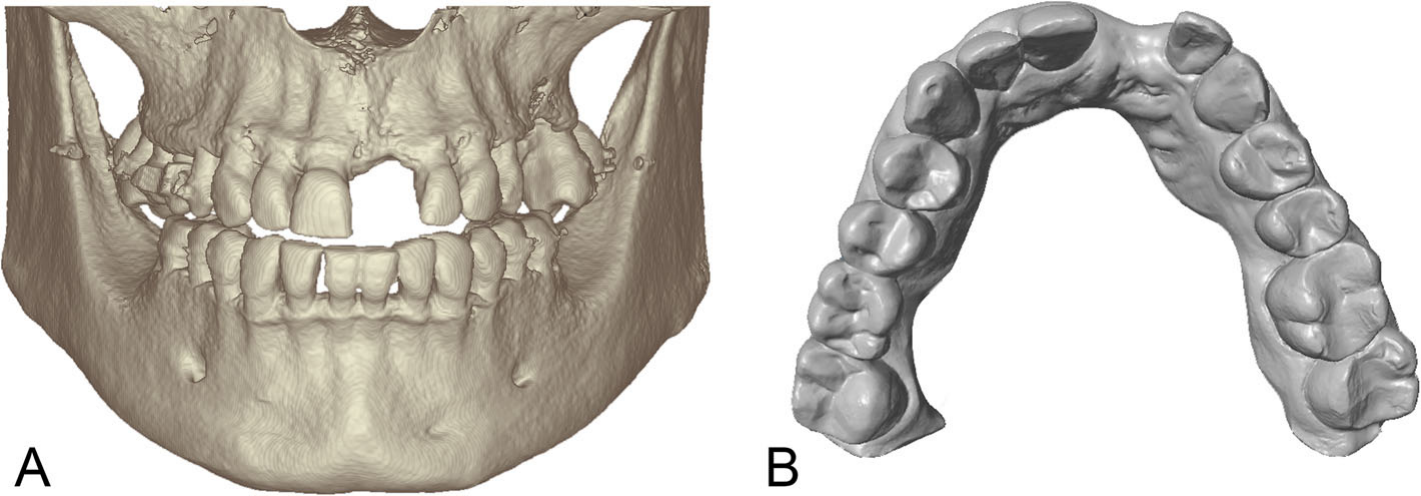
Display of data import options. All systems could import (CB-)CT data displaying the alveolar bone in the implant region (a) and virtual stone casts representing tooth and mucosa surfaces (b)
